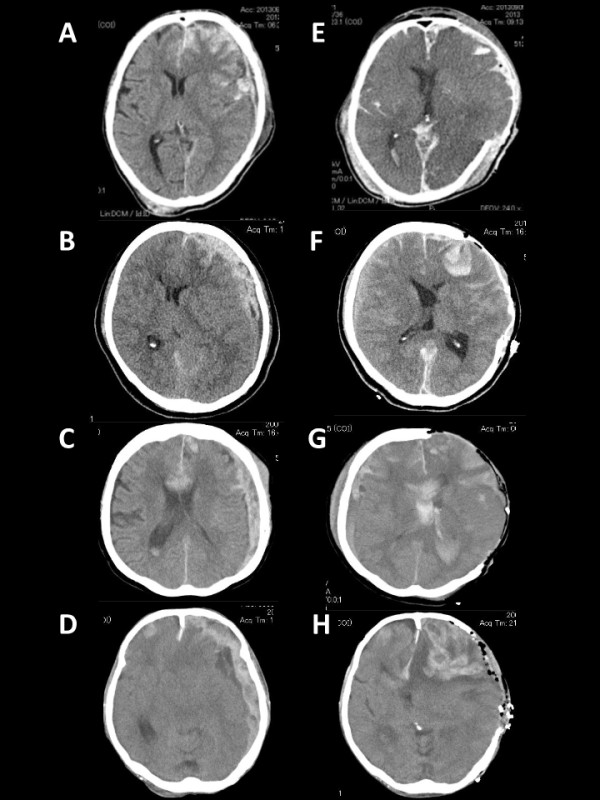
Pre- (A, B, C, D) and postoperative (E, F, G, H) computed tomography (CT) scans of four left acute traumatic subdural hematoma (ASDH) patients with contusion, all of whom sustained fatal brain swelling. A, E: a 64-year-old man with Glasgow Coma Scale (GCS) score of 3; B, F: a 73-year-old woman with GCS score of 6; C, G: a 71-year-old woman with GCS score of 11; D, H: a 68-year-old man with GCS score of 4. All postoperative CT scans were obtained within 24 h of surgery.
